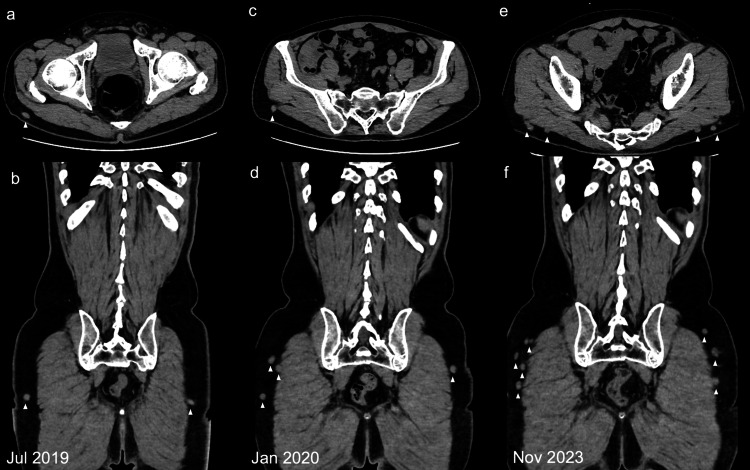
Follow-up CT images Follow-up C) images after 3 (a, b), 10 (c, d), and 60 (e, f) lanreotide depot injections (a, c, e: axial views; b, d, f: coronal views). Persistent nodular lesions (arrows) in subcutaneous nodules on the buttocks over time. CT, computed tomography
